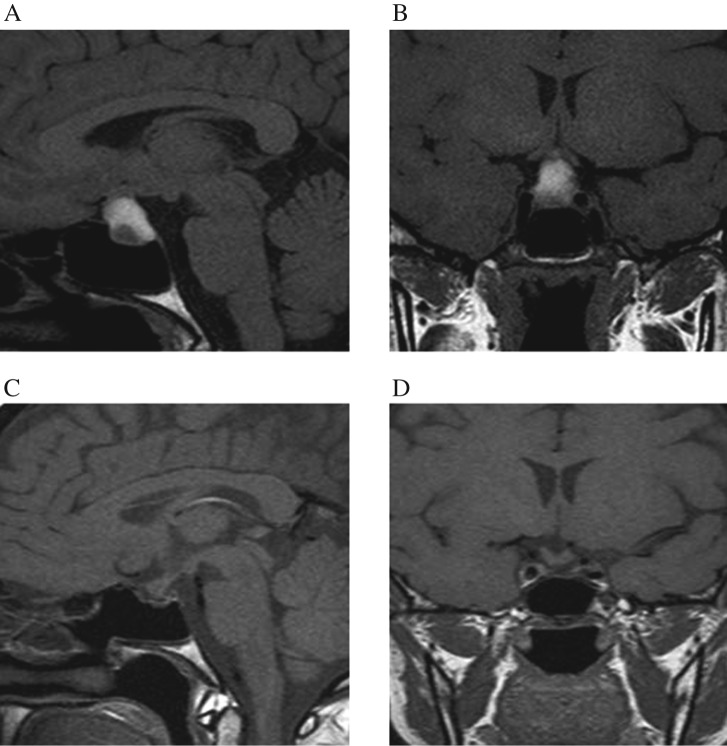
(A and B) Preoperative nonenhanced MRI: an extensive intrasellar lesion expanding into suprasellar region was found, with signs of bleeding, compression and optic chiasm displacement. (C and D) Postoperative nonenhanced MRI under meprednisone treatment showed significant reduction in sellar mass size.
